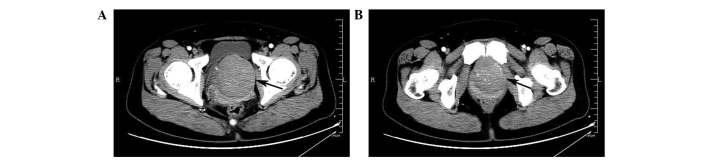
Contrast-enhanced computed tomography showing the presence of the tumor (A) compressing the bladder and uterine and (B) behind the pubic symphysis. Arrows indicate the tumor.
